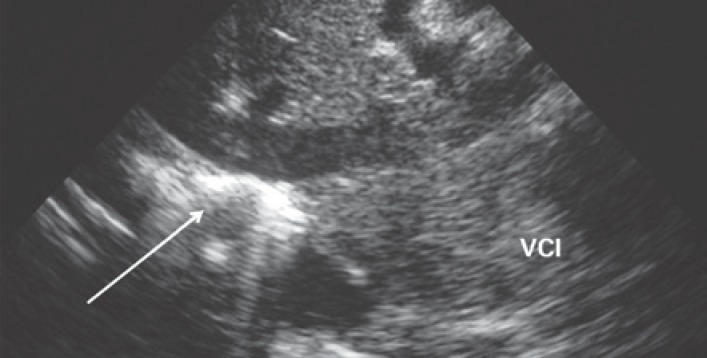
Pneumothorax. Lung sliding is absent (dynamic sonography); therefore, the pleural line (arrow) does not move as the patient breathes in and out VCI: inferior vena cava.
